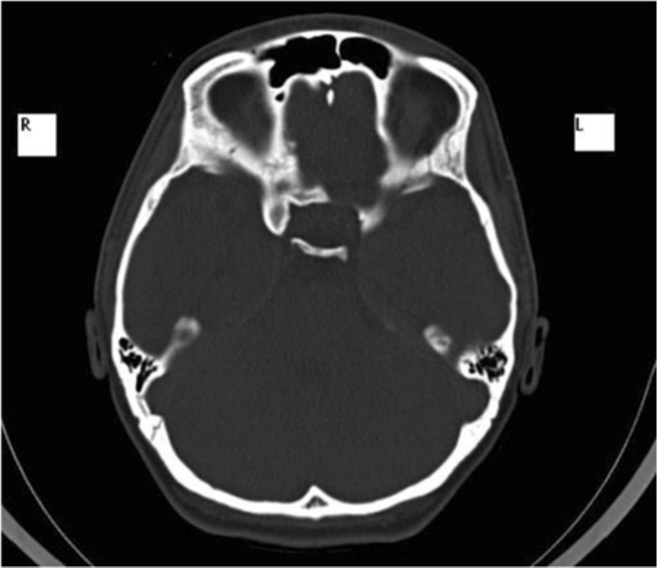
High-resolution computed tomography scan, axial projection.
